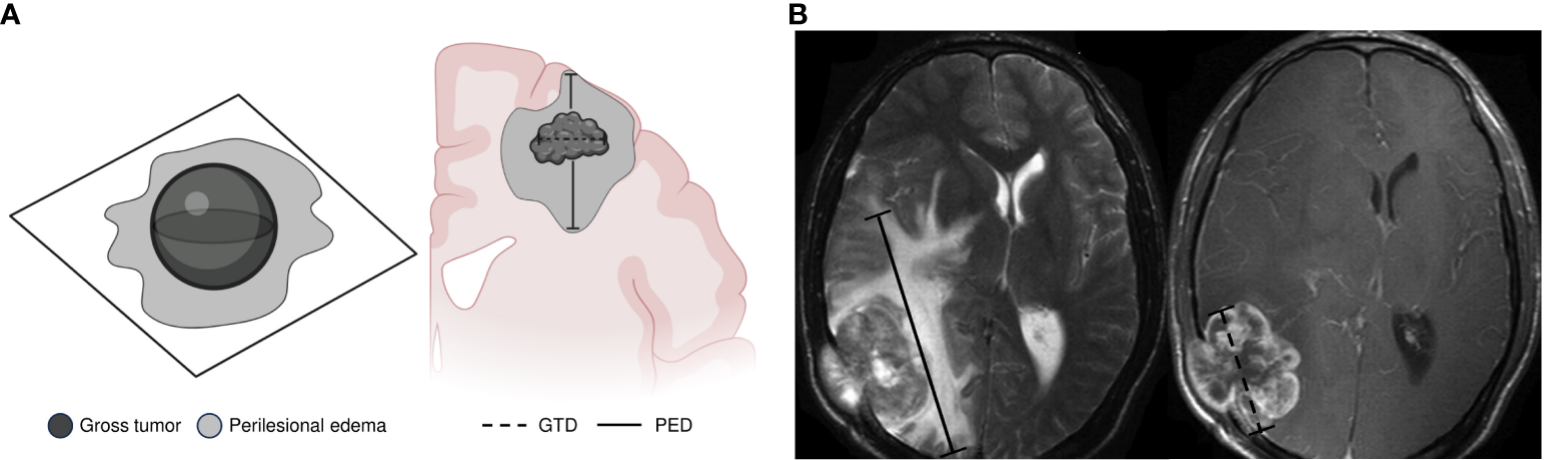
Method used for measuring peritumoral edema extent. We selected the most representative images based on the tumor’s maximum diameter and maximum edema extent on midplane axial, sagittal, or coronal sections. (A) Gross tumor (GT) was established as the sum of the maximum diameter (mm) of the three most representative T1-weighted gadolinium-enhanced lesions. Perilesional edema (PE) was defined as the sum of the maximum diameter (mm) at the perilesional hyperintense area on a T2-weighted or FLAIR MRI sequence. (B) Examples of perilesional edema diameter (PED) measurement on MRI FLAIR sequences (axial) and gross tumor diameter (GTD) measurement on MRI T1 gadolinium-enhanced scans (axial). GT was established as the sum of the maximum diameter of the three most representative lesions. PED was defined as the sum of the maximum diameter of the perilesional hyperintense area. Dotted black lines indicate the maximum GTD in millimeters (mm). Solid black lines indicate the maximum PED in mm. The PED/GT ratio was calculated by dividing the PED maximum extent by the maximum tumor diameter. GT, gross tumor; GTD, gross tumor diameter; PED, perilesional edema diameter.
